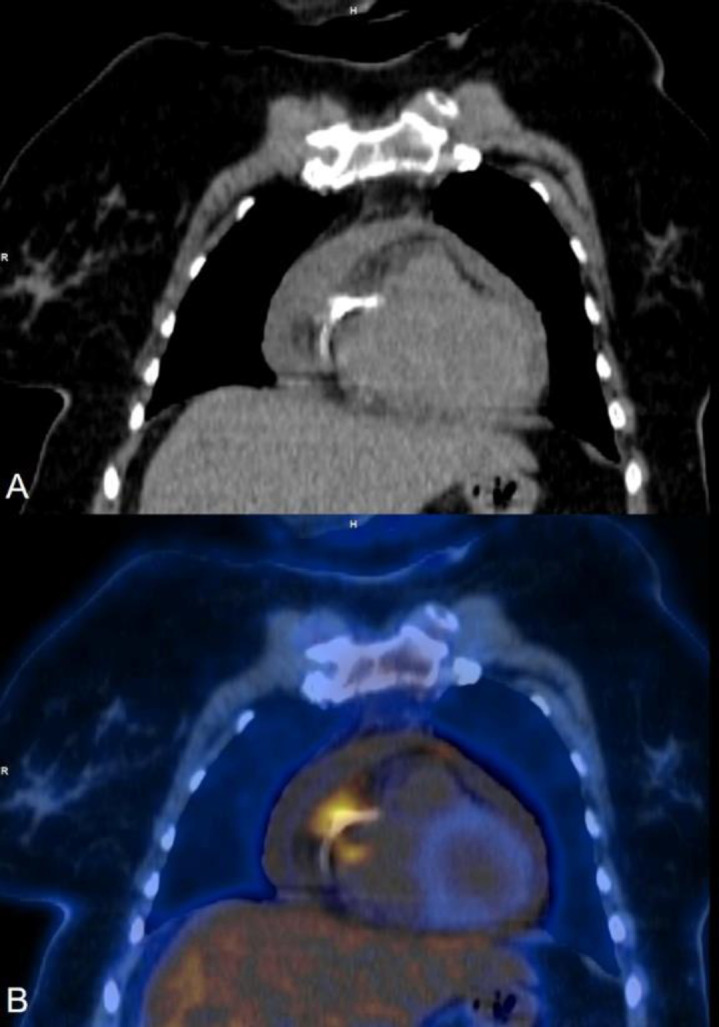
Coronal section (A) CT and (B) fused FDG PET CT images showing focal abnormal increased FDG uptake (SUV_Max_: 4.5) along the RCA stent
